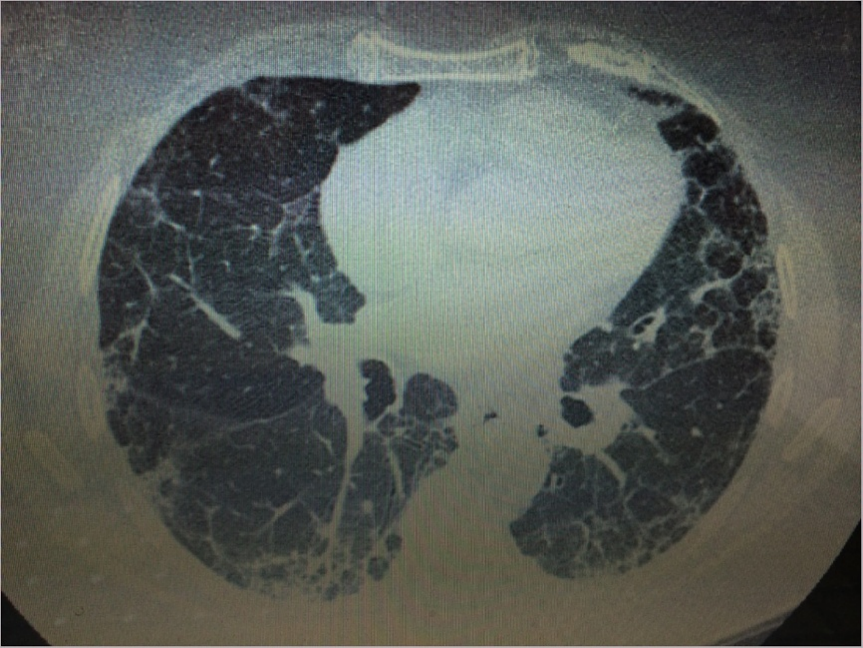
HRCT scan demonstrating PF with UIP.
